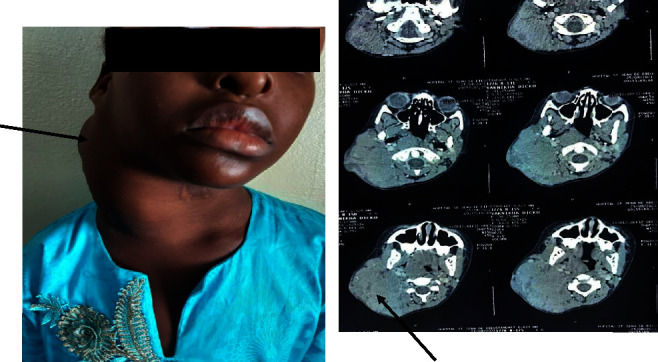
Diagnostic appearance : right laterocervical mass (black arrow) at clinical examination (a) and at CT cervical scan (b).
